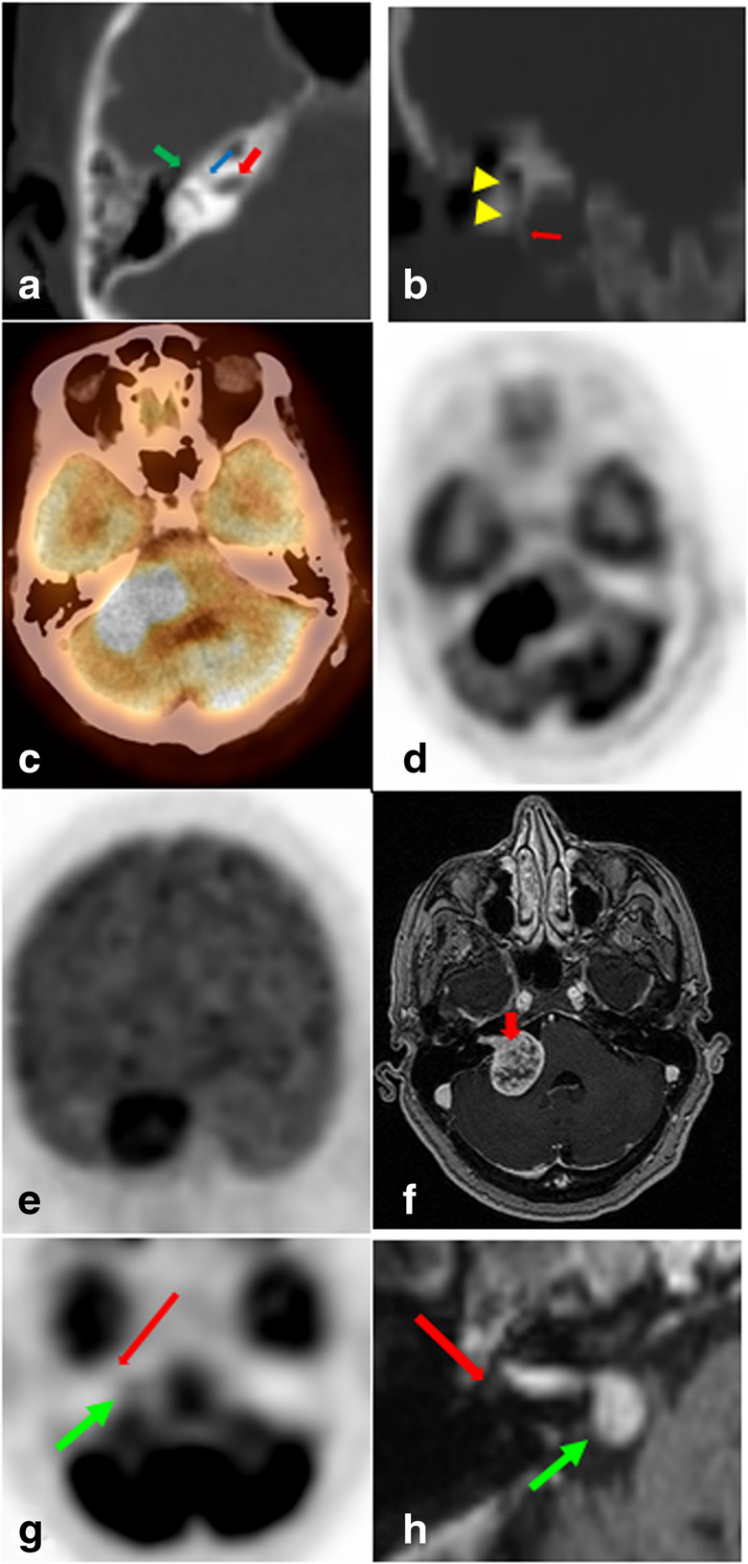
Axial (a) and coronal (b) low resolution CT part of PET/CT. a On the axial view the internal auditory canal parts of CNVII and VIII (red arrow), as well as the labyrinthine (bluearrow) and tympanic parts (green arrow) of CN VII can be visualized. b On the coronal view,the styloid process (red arrow) can be used as a landmark to visualize the stylomastoid foramen,containing the stylomastoid part of CN VII (arrow heads). c–f A 62 year-old-female with rightsided hearing loss. Axial fused/non fused FDG PET/CT (c, d) and coronal Maximum Intensity projection (MIP) (e) images showing an intensely hypermetabolic right cerebellopontine angle lesion (CPA) lesion. Given patient history of hearing loss, this was consistent with vestibular schwannoma, rather than a meningioma. Axial contrast enhanced T1 WI MRI (f) showing the classic heterogeneously enhancing “ice cream cone” mass extending into the right internal auditory canal (IAC) (arrow), consistent with vestibular schwannoma, which was confirmed pathologically. g, h A 71-yearold female patient with history of worsening right sided hearing loss, unsteadiness, and neck pain. Axial unfused PET/CT images (g) and contrast enhanced T1 WI MRI (h) show a right CPA enhancing lesion with mild focal FDG activity (SUVmax 3.0) (green arrow), extending along the IAC, labyrinthine and tympanic segments of CN7 (red arrow) confirming that this is a facial nerve schwannoma rather than the more common vestibular nerve schwannoma
